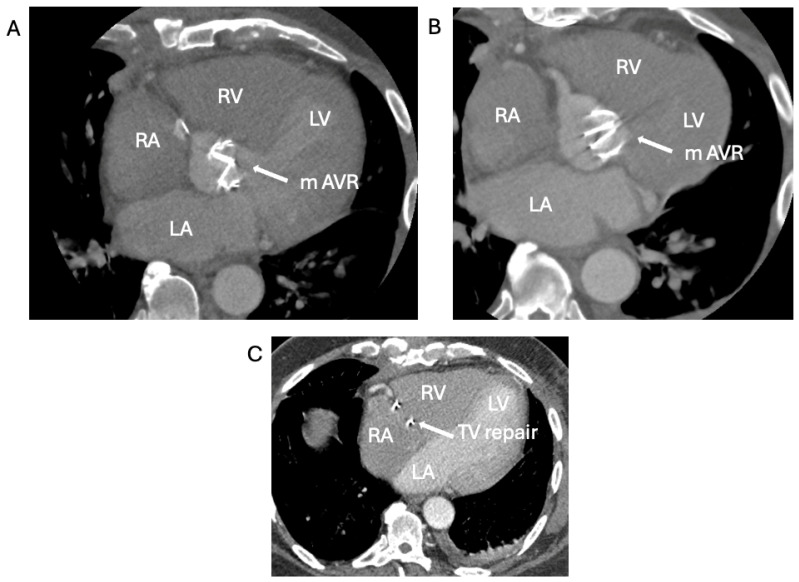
CT images of mechanical AV replacement and TV annuloplasty ring. (A): mechanical aortic valve in diastole. (B): mechanical aortic valve in systole. (C): TV annuloplasty ring.
